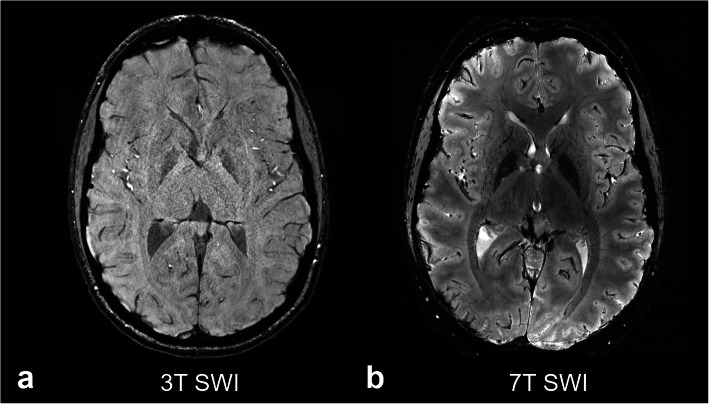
Comparison of susceptibility-weighted imaging (SWI) using 3-T and 7-T magnetic resonance imaging. Axial SWI images are shown at the level of the basal ganglia in a healthy individual acquired at 3 T (a) and 7 T (b). At higher field strength, the shorter T2* relaxation times result in the greater SWI sensitivity to magnetic susceptibility differences in the blood vessels and mineralization in the basal ganglia, particularly in the globus pallidus
